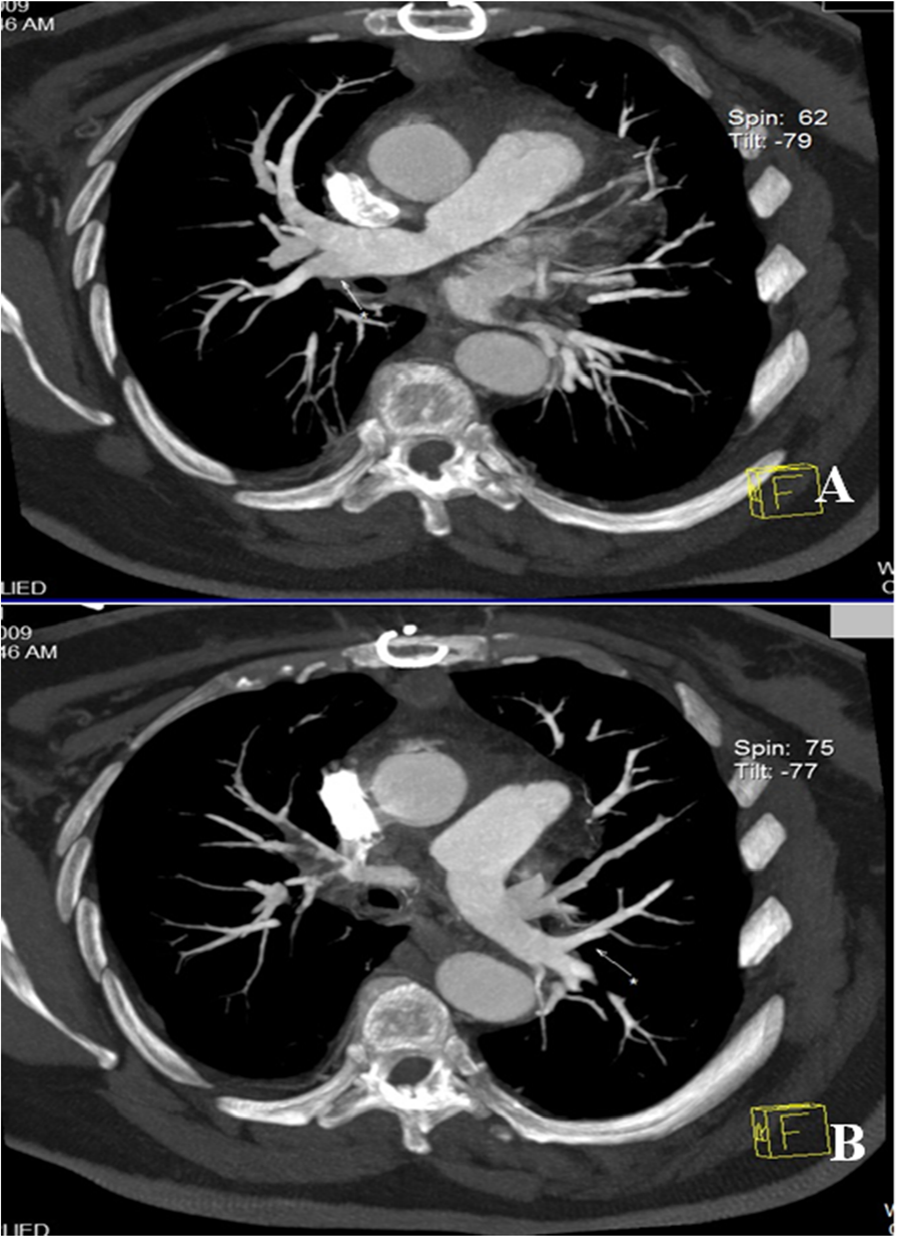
Post-operative imaging studies. (A) Post-operative contrast-enhanced computed tomographic scan shows total removal of the thrombus on the right pulmonary artery. (B) Post-operative contrast-enhanced computed tomographic scan shows total removal of the thrombus on the left pulmonary artery.
